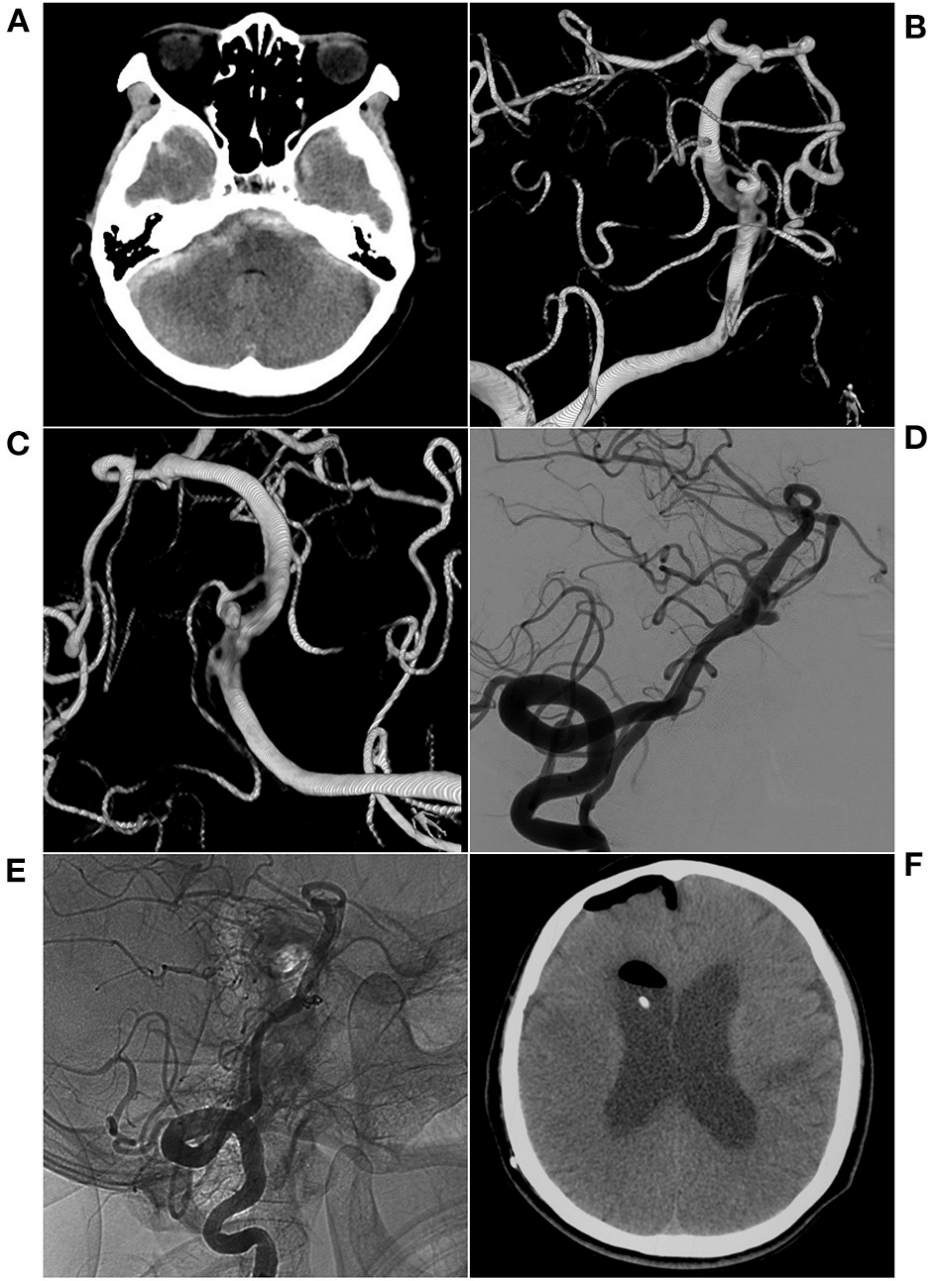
EVT with single coiling of an aneurysm in the fenestration. (A) CT shows subarachnoid hemorrhage in the anterior brainstem; (B,C) 3D reconstructed DSA shows two fenestrations in the inferior BA and two aneurysms in the superior fenestration. (D) Preoperative VA-DSA shows a large aneurysm. (E) Postoperative VA-DSA images show a large aneurysm with simple coiling, MRRC Grade I. (F) The patient had hydrocephalus after EVT and underwent ventriculoperitoneal shunting at the 10-month follow-up. BA, basilar artery; CT, computed tomography; DSA, digital subtraction angiography; EVT, endovascular therapy; MRRC, Modified Raymond-Roy Classification; VA, vertebral artery.
